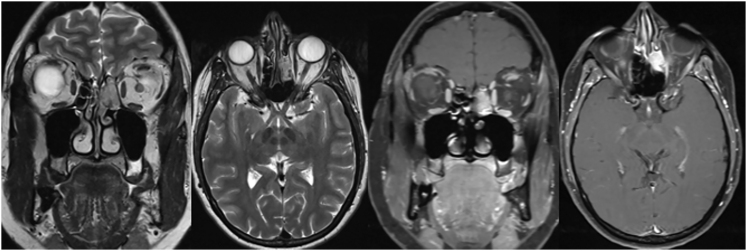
Preoperative MRI shows an intensely arterialised expansive tumour without locally invasive characteristics (21 × 20 × 10.5 mm). It is situated at the left anterior ethmoidal labyrinth, reaching the Onodi cells, then extending under the ethmoidal fovea and inside the left middle concha in contact with the nasal septum. There is no modification of the anterior floor of the skull base.
